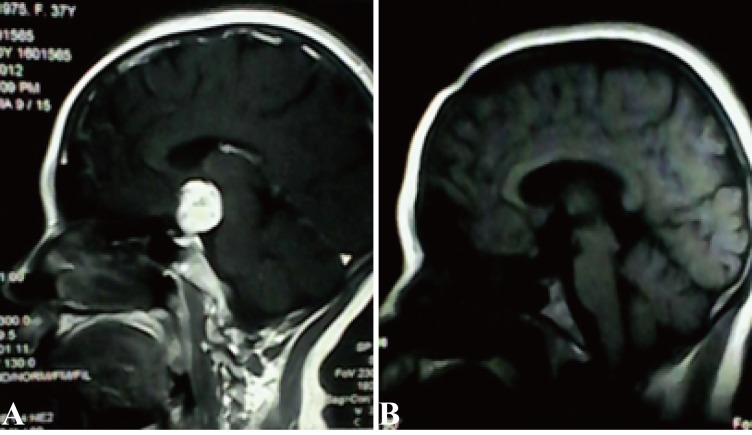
MRI images of case 1. (A) Preoperative MRI image revealing a suprasellar round mass. (B) MRI image at the 21-month follow-up exhibiting no recurrence of the tumor. MRI, magnetic resonance imaging.
